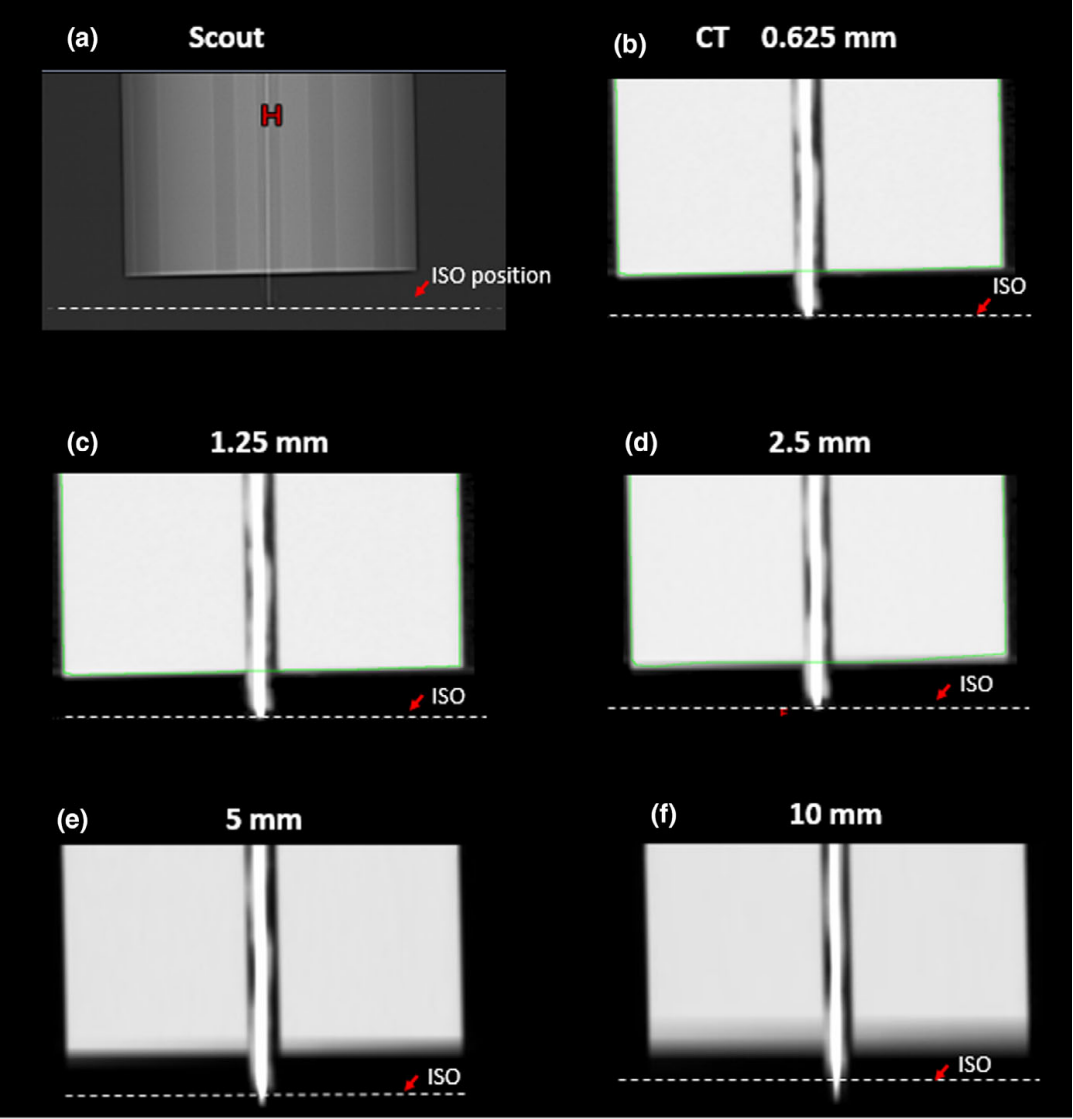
Needle tip shown in a) anterior‐to‐posterior scout image, and b)‐f) CT images with different slice thicknesses. Dash line (ISO) shows the laser position which was aligned to the real needle tip during the setup process. Green lines showed automated contours for body.
